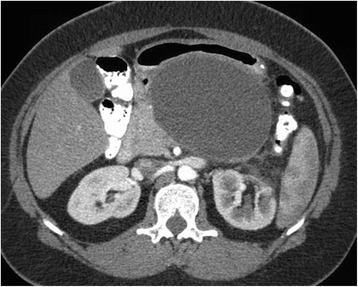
Duodenal compression (axial CT image)
